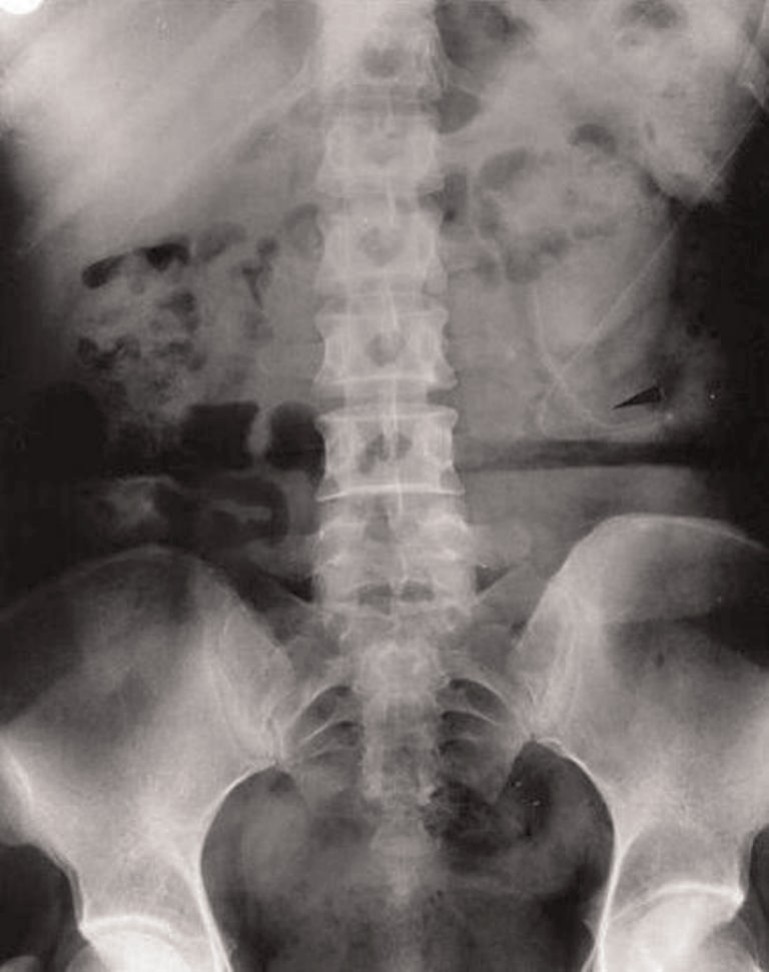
The abdominal X-ray film revealed the course of the peritoneal catheter (arrow).
